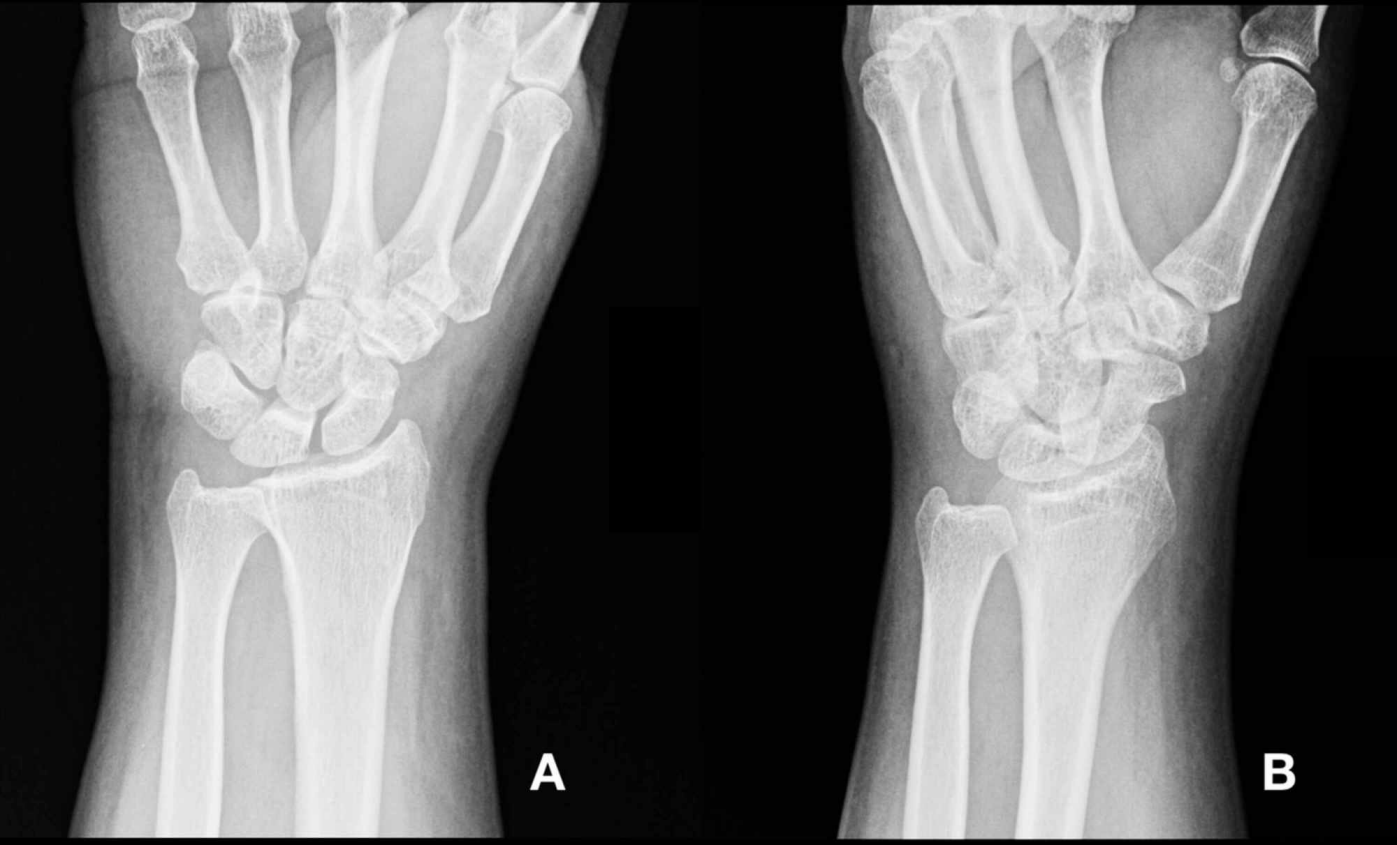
Plain radiograph of the left hand showing mild soft tissue swelling of the wrist. Joint spaces are well maintained. No fracture, dislocation, periosteal reaction, or osteolysis is noted. (A) Anteroposterior view. (B) Oblique view.
